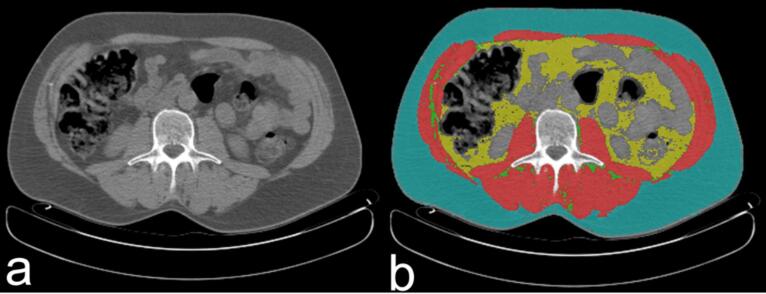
Body Composition Assessment on CT-Scan Slice.
